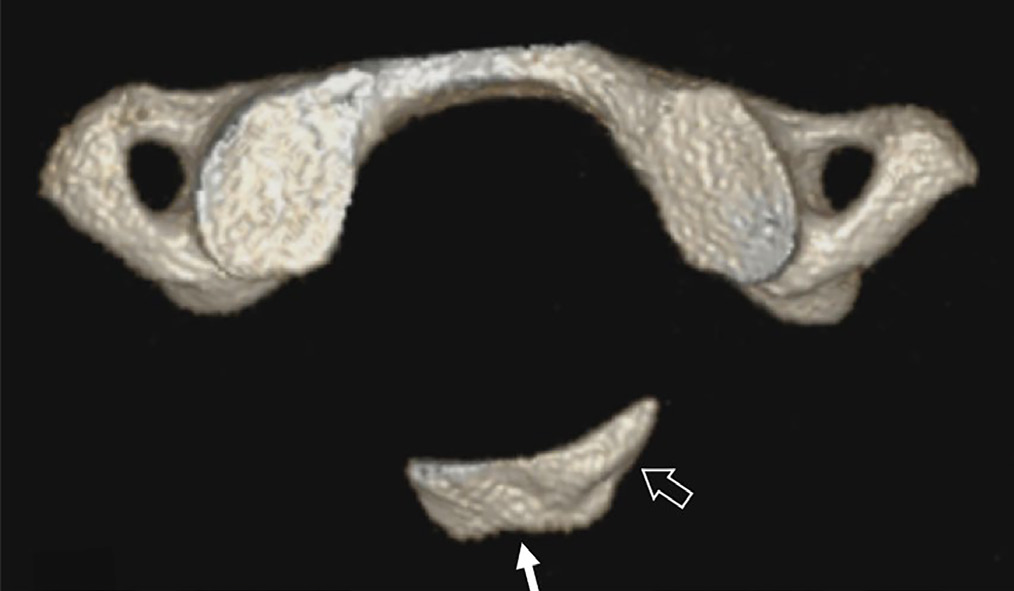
3D reformatted CT static image showing hypoplasia of the posterior arch of atlas with a persistent posterior tubercle (arrow) contiguous with a small left paramedian posterior arch remnant (open arrow), compatible with a Currarino type D posterior arch anomaly.
